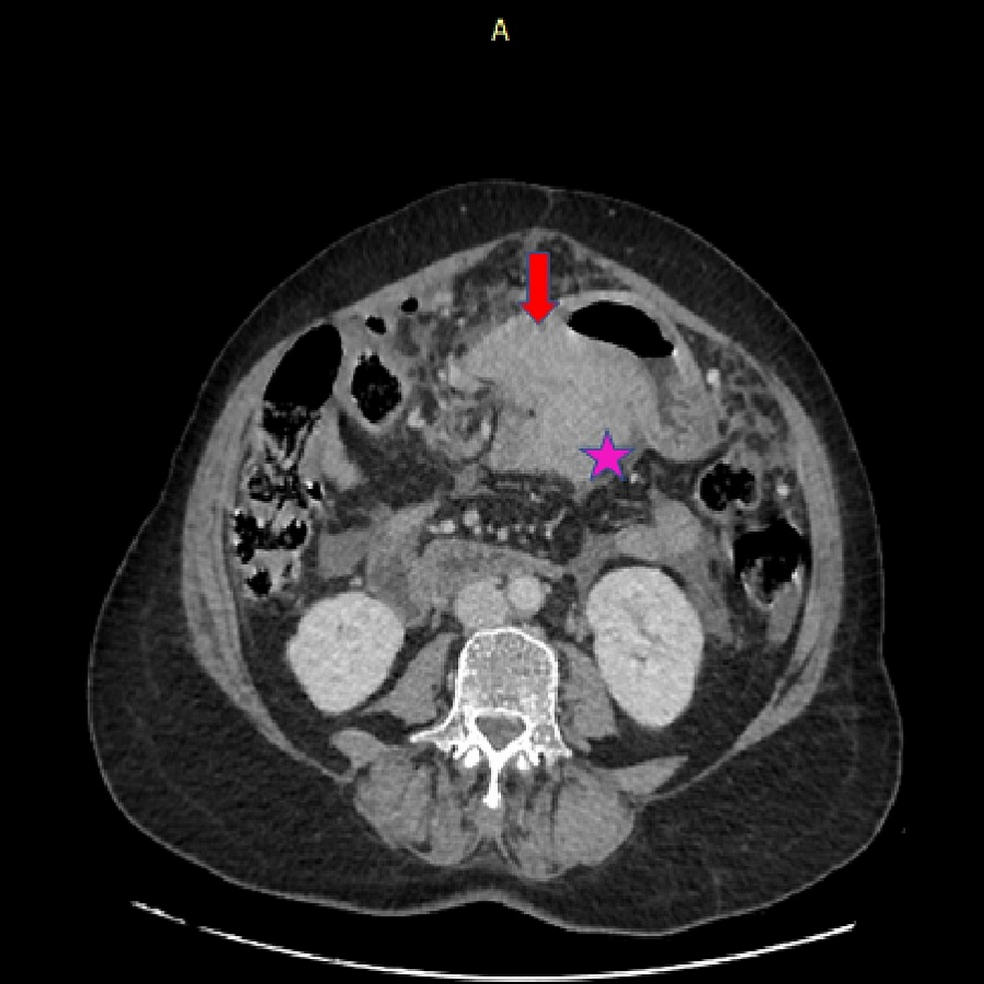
Image of axial section of CECT abdomen showing gastric wall thickening in the antropyloric region (red arrow) with enlarged perigastric lymph nodes (pink star) CECT - contrast-enhanced computed tomography
